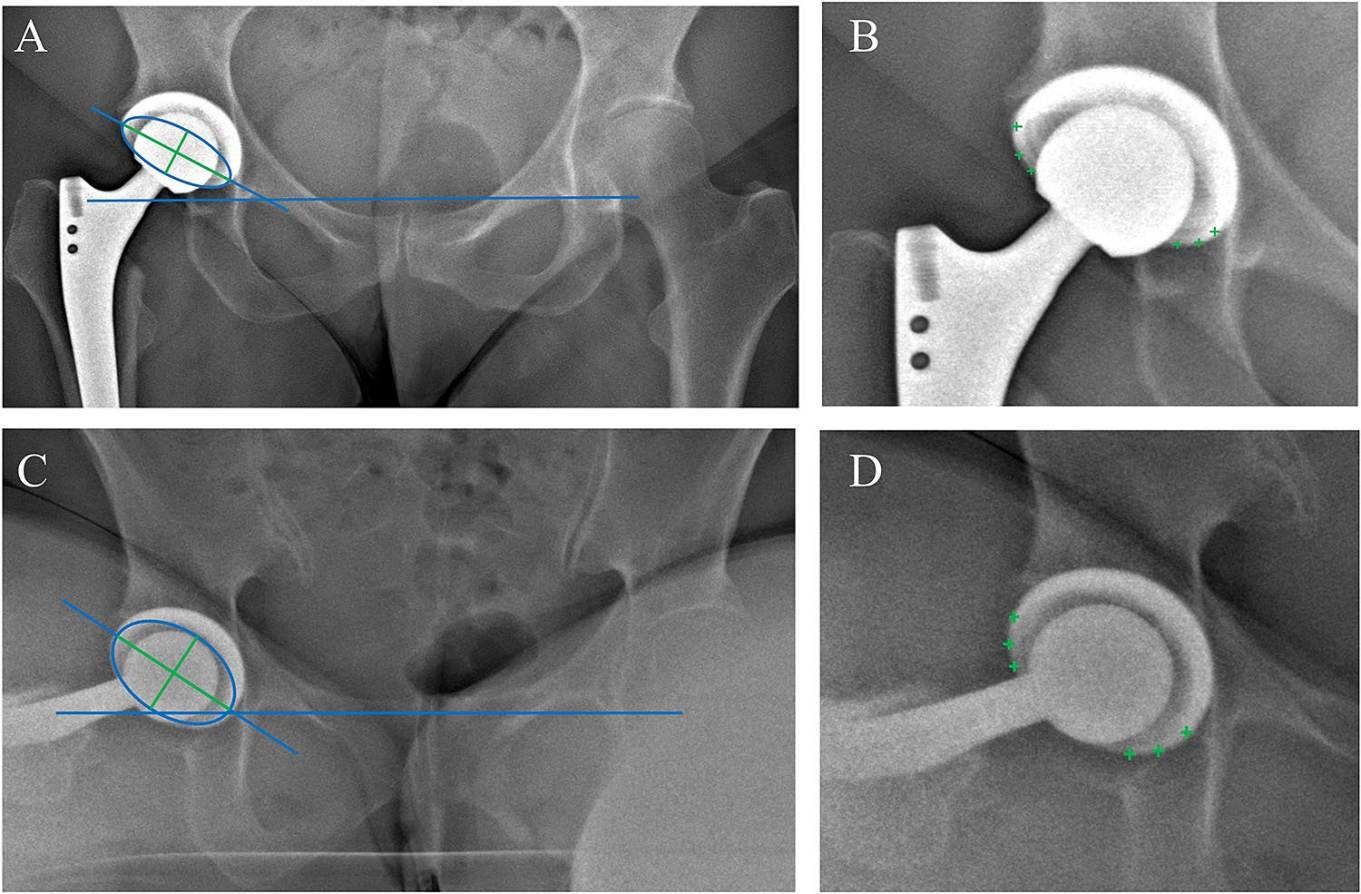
Method of the measurement of acetabular cup anteversion and inclination in a.p. standing (A, B) and sitting (C, D) radiographs by defining the rim of the cup (green + in C, D), and building an ellipse (blue circle; A, C) with a long and short axis (in green). Blue horizontal line is defined as interteardrop line. Combination of green and blue line displayed the long axis of the ellipse. Anteversion is defined as arc sine (short axis/long axis) of the ellipse and inclination as the angle between interteardrop line and long axis of the ellipse
